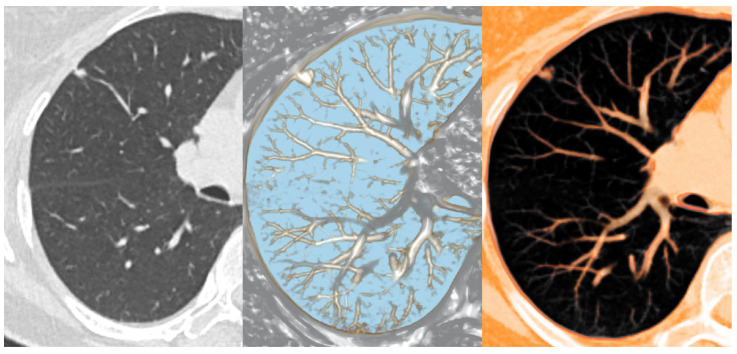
Thin-slab 1 mm axial CT view (left), nodule detection view of MPVR (middle), and PSN view of MPVR (right) from the CT data set of a 60-year-old patient with a pulmonary nodule predominantly composed of a solid component.
